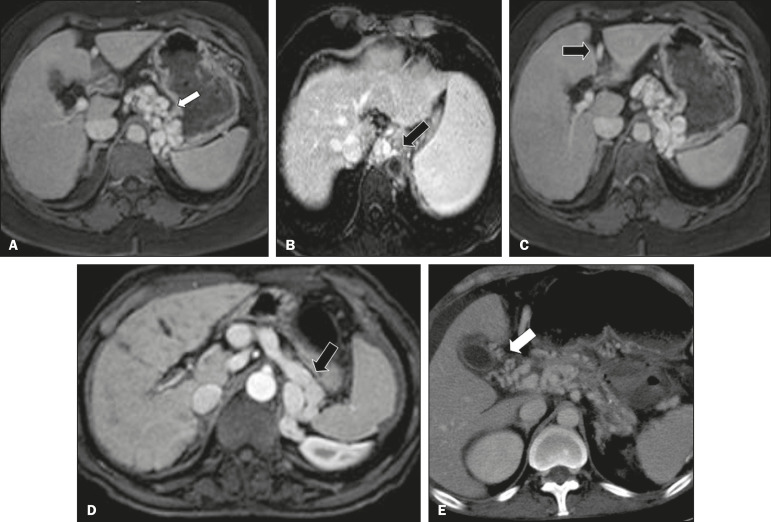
A: Gastroesophageal varices. Axial contrast-enhanced CT scan showing dilated vessels at the lesser curvature and at the gastroesophageal junction (arrow). B: Contrast-enhanced MRI showing paraesophageal varices, presenting as dilated vessels around the distal esophageal portion (arrow). C: Contrastenhanced axial T1-weighted MRI scan showing recanalization of the paraumbilical veins, the reopening of the veins occurring in the round/falciform ligament (arrow). D: Contrast-enhanced MRI of a splenorenal shunt. There is communication between the splenic vein and the left renal vein through serpiginous, dilated vessels (arrow). E: Axial contrast-enhanced CT scan showing dilated and tortuous vessels in the gallbladder wall (arrow).
